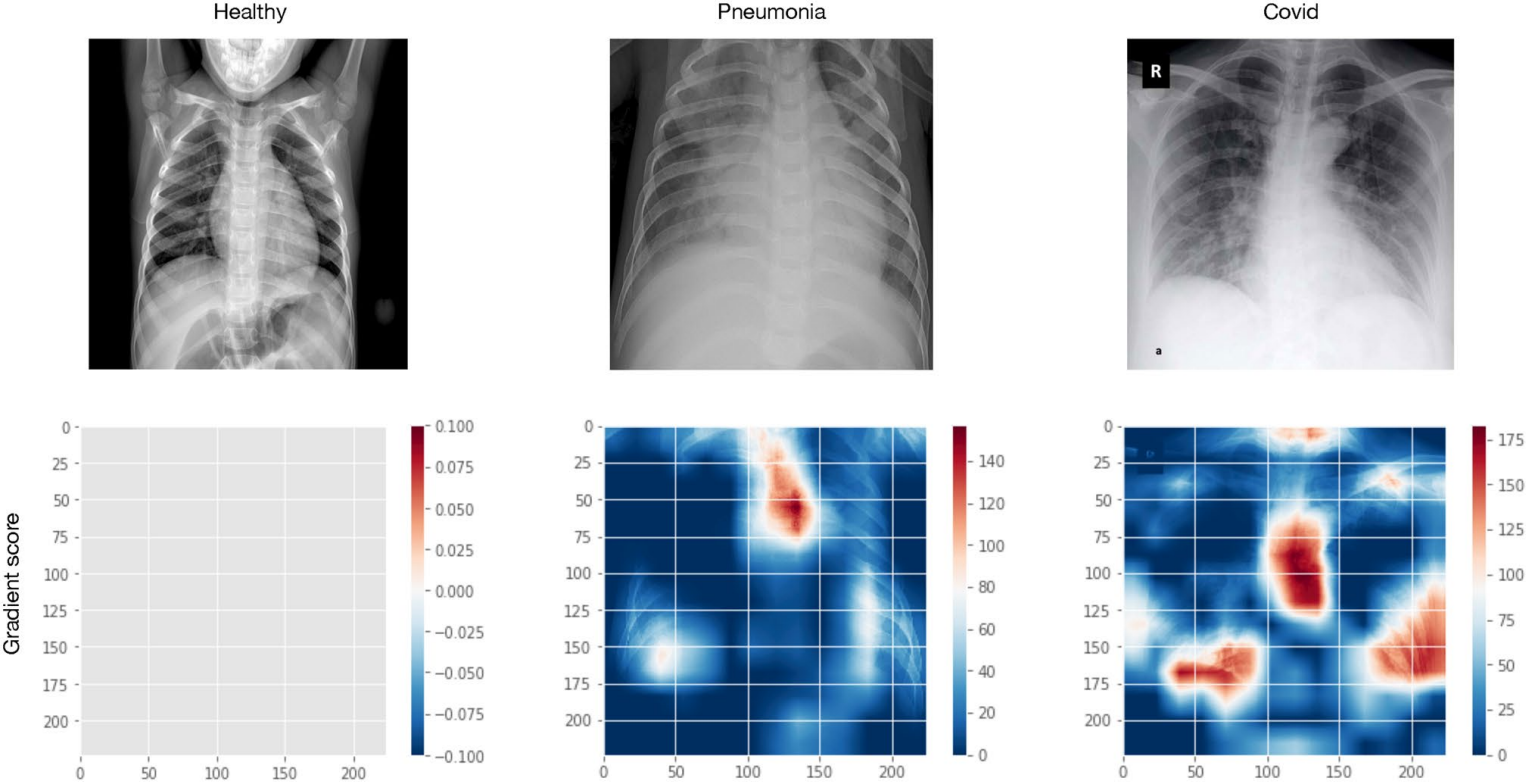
Tuples of X-ray radiographs and their feature space highlighted by Grad-CAM maps. It shows a sample of a healthy individual (left), a patient diagnosed as positive for pneumonia disease (centre), and a patient diagnosed positive for COVID-19 (left)
